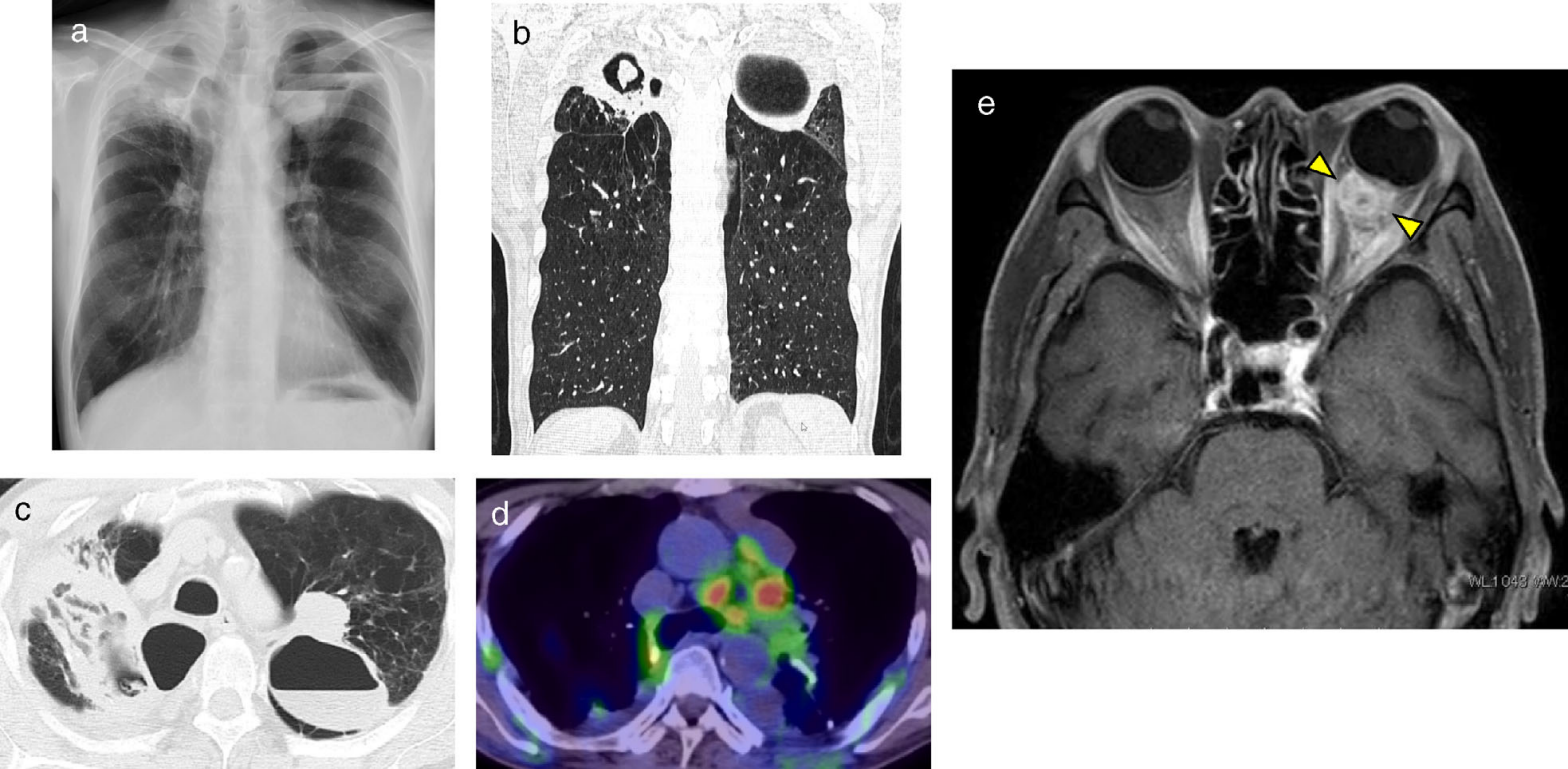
(a) Chest X‐ray from previous hospital. (b, c) Chest computed tomography (CT) revealed cavity formations in both upper lobes. (d) Positron emission tomography‐computed tomography (PET‐CT) scan showed high level uptake of FDG in the mediastinal lymph nodes. (e) Magnetic resonance imaging (MRI) of the head revealed an 11 mm nodule in the left choroid (arrows).
